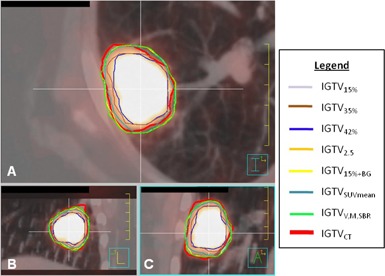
PET/CT images of tumor 13 with internal target volume (ITV) contours: (a) transverse, (b) sagittal, and (c) coronal. ${\text{ITV}}_{\text{CT}}$, shown in red, was delineated on the maximum intensity projection. Other ITVs were delineated on PET using methods described in Table 1. The VMSBR model (${\text{ITV}}_{\text{VMSBR}}$) is shown in green.
